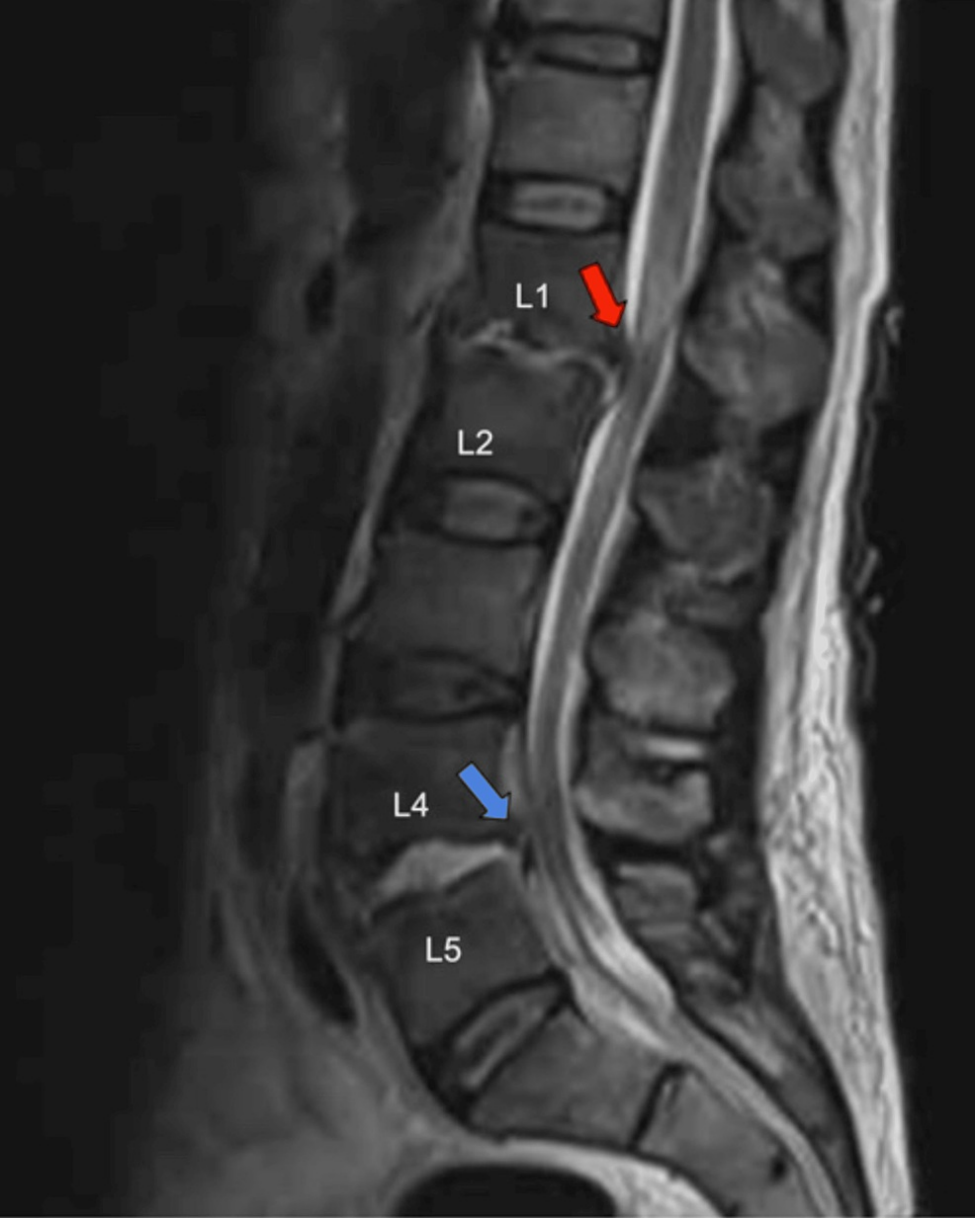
T2-weighted sagittal view revealing L1-L2 and L4-L5 discitis/osteomyelitis with associated extensive phlegmonous enhancements (red arrow) and a thin ventral abscess at L4-L5 (blue arrow).
